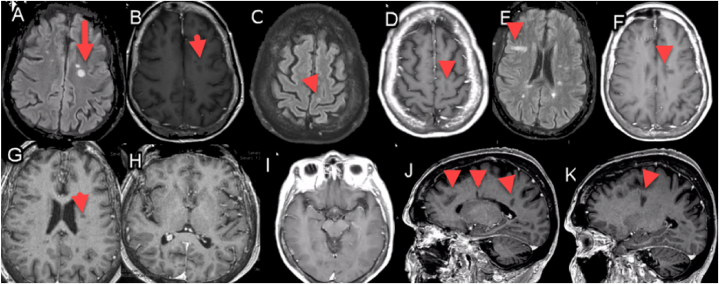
Legend –Representative neuroimages from most recent brain MRI demonstrating stable findings for an approximately 3-year period. Representative neuroimages from most recent brain MRI demonstrating stable findings for an approximately 3-year period. A: Axial flair showing prominent lesions. B: Axial T1 weighted neuroimaging with gadolinium showing no enhancement C: Axial flair with gadolinium showing a small cortical lesion along with D: T1 weighted imaging in a similar section as C. E: Additional axial flair MRI imaging at the level of the lateral ventricles. F: T1 with gadolinium enhanced MRI showing no definite enhancement along with additional sections at G and H highlighting a region at the level of the basal ganglia. I: Highlights an axial T1 with gadolinium section highlighting the preservation of mesial structures (hippocampi and temporal lobes) and J and K: sagittal T1 MRI selections with gadolinium highlighting lesions in periventricular and deep white matter locations.
